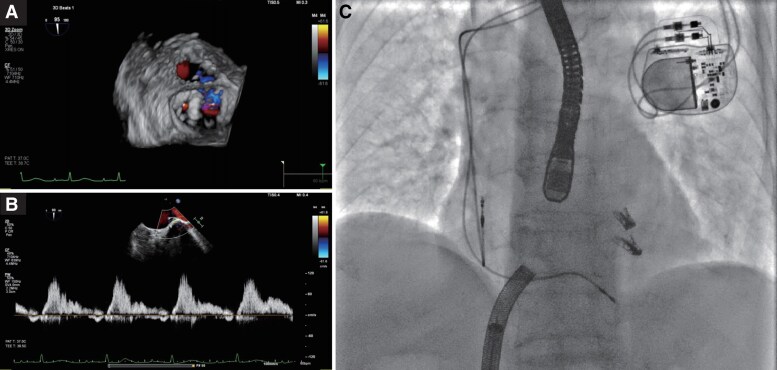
Postprocedural assessment after two clips implantation (*A*) trace residual mitral regurgitation after procedure. (*B*) Reduced pulmonary vein flow reversal. (*C*) DSA showing the relative position and orientation of the two clips, with the inferior clip (the second) deployed at a large opening angle. TEE, transoesophageal echocardiography.
